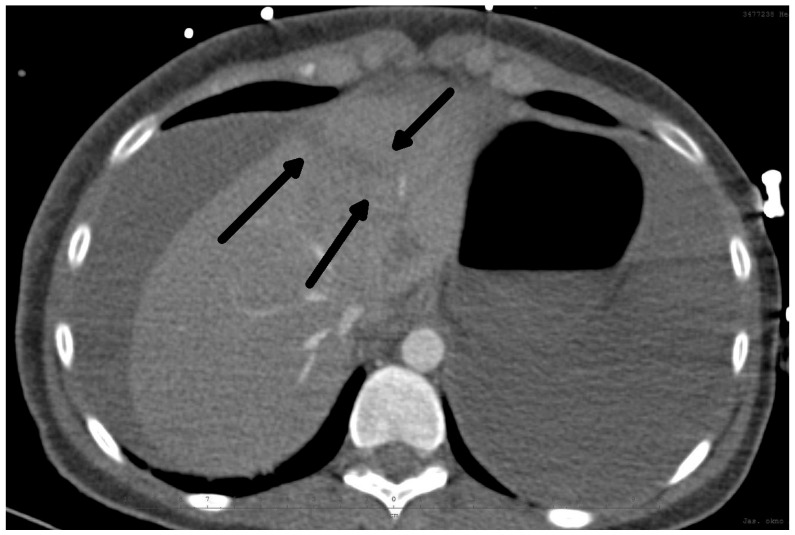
CT scan: Rupture of the liver along falciform ligament (arrows) and hemoperitoneum transverse view.
